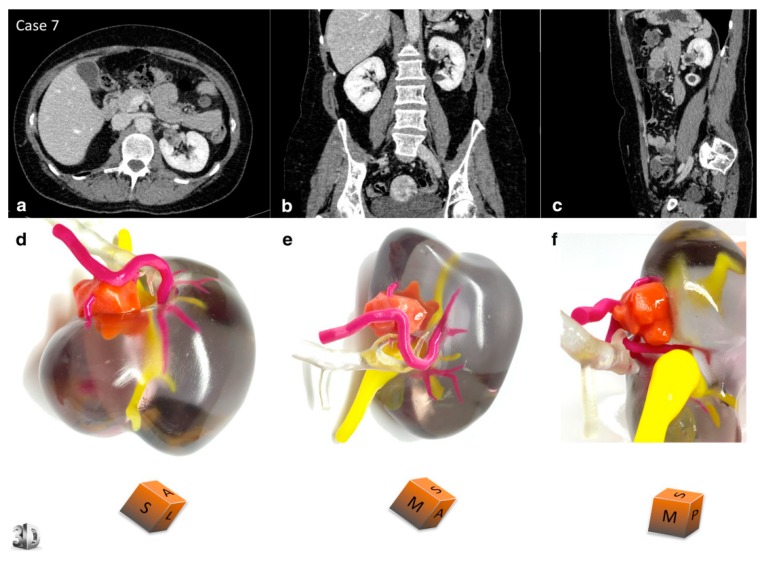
3D-printed model of a 53-year-old female with a renal tumour at the interpolar region of left kidney. Comparative views of the CT scan at the nephrographic phase ((a) axial, (b) coronal, and (c) sagittal planes) and corresponding views of the physical model ((d) superior view, (e) median view, and (f) median view). The cubes show the 3D-printed model orientation in space (I = inferior face, A = anterior face, L = lateral side, S = superior face, P = posterior face, M = median side). The patient underwent a left partial nephrectomy for a 21 × 15 × 15 mm angiomyolipoma. Description of colour corresponding to different renal structures and tumour is the same as in Figure 4. Reprinted with permission from Bernhard et al. [6].
